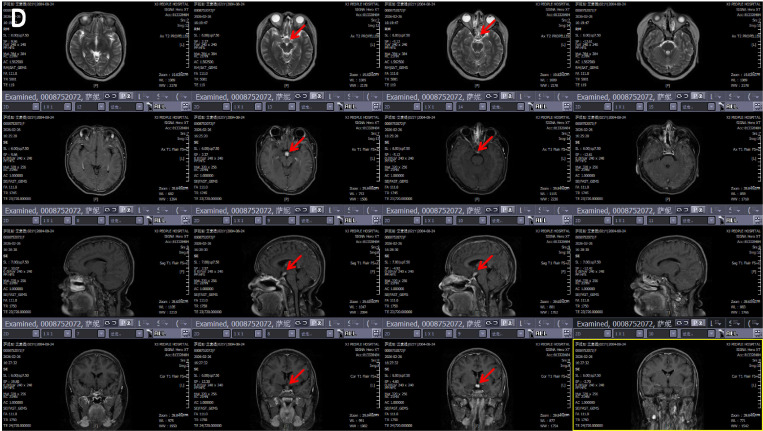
Cranial MRI of the hypothalamic–pituitary region after 6 cycles of chemotherapy. Left: T1-FLAIR sequence; Right: T2-FLAIR sequence. No significant change in the residual pituitary lesion is seen compared with pre-chemotherapy imaging.
